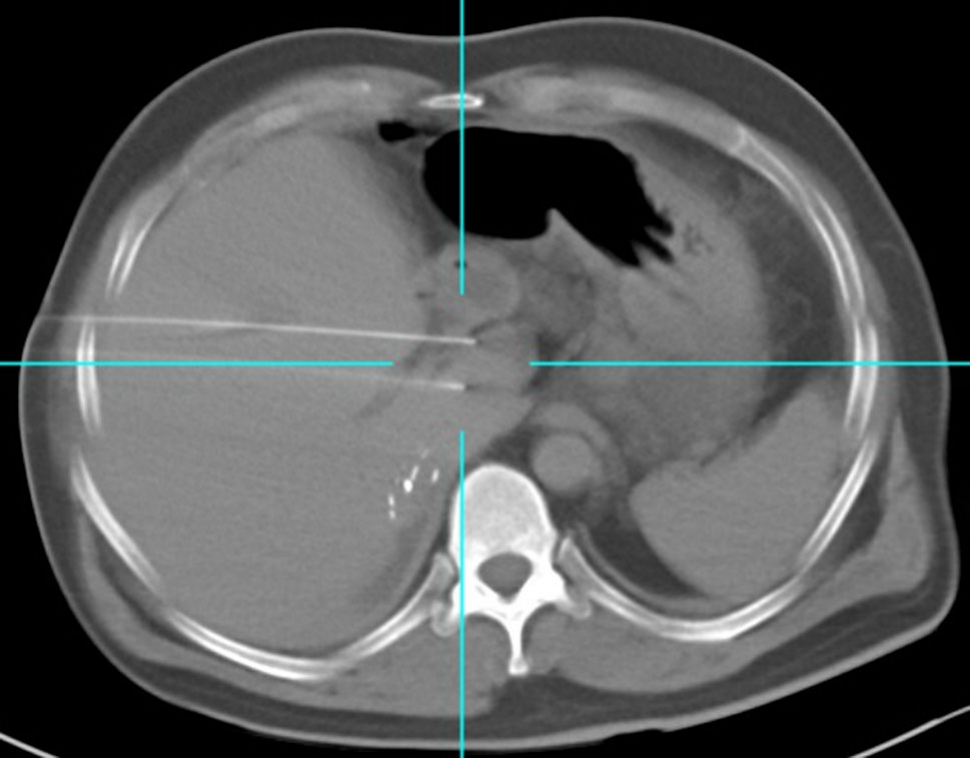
CT demonstrating  parallel IRE electrode position for treatment (third needle not shown).
